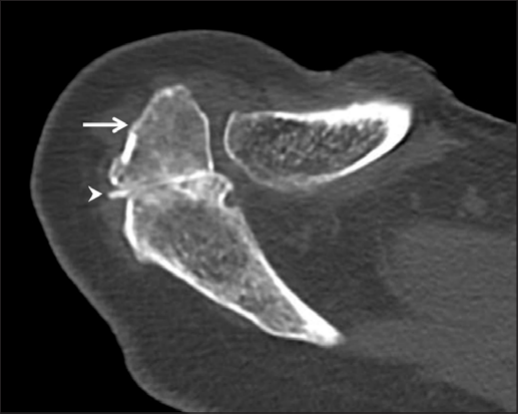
Os acromiale. Axial CT arthrography through the acromioclavicular joint demonstrates an os acromiale (arrow) with synchondrosis (arrowhead). An os acromiale should not be confused with a fracture fragment and the synchondrosis with a pseudarthrosis. In this case, there is a communication between the synchondrosis and the glenohumeral joint (with high-density contrast penetration), which is not normal. Subchondral cystic changes are also seen in the zone of pseudarthrosis.
